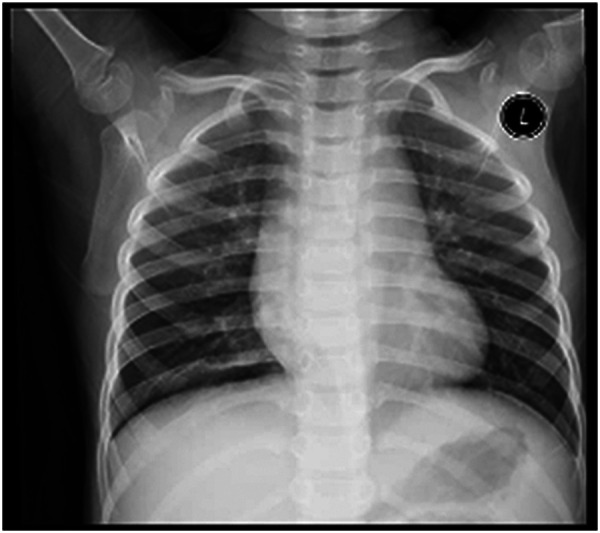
Chest x-ray findings six months into the course of the illness.
